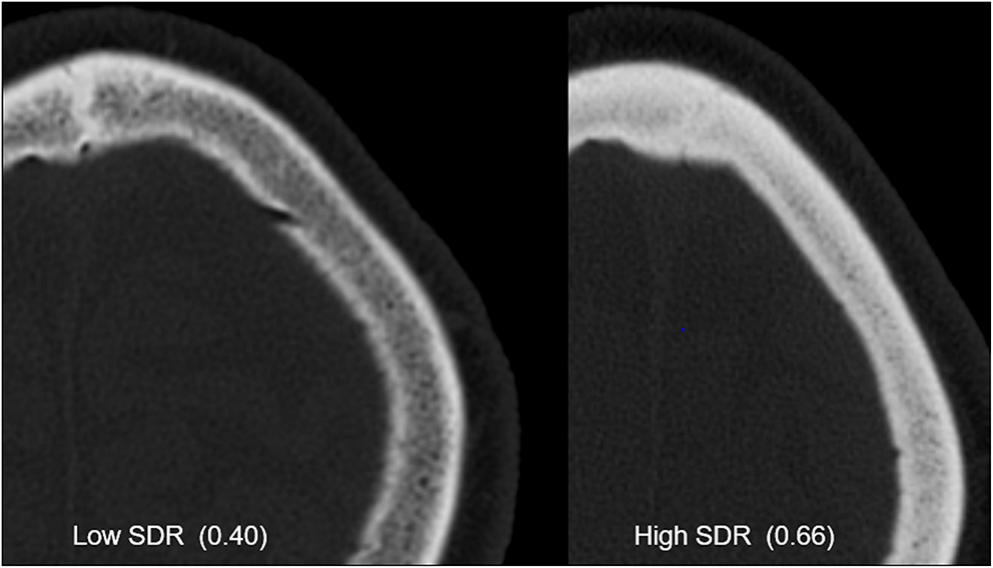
CT images from patients with low and high skull density ratios (SDRs). Both images are windowed the same way, and the skull with high SDR clearly shows increased Hounsfield units.
